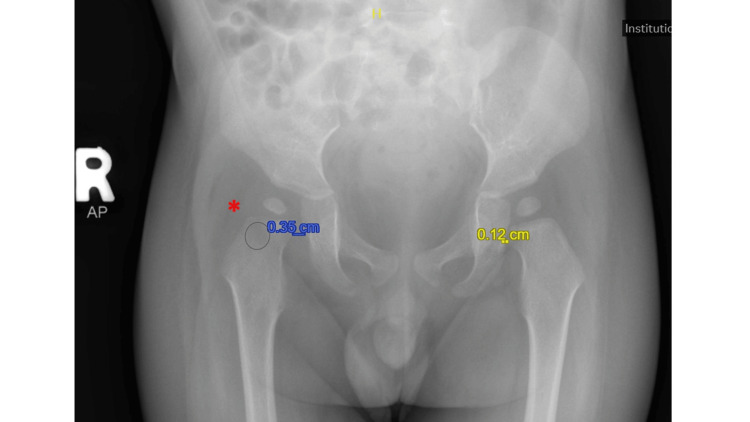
Anteroposterior radiograph of the pelvis. The image demonstrates widening of the right hip joint space, marked with red asterisk with metaphyseal rarefaction of the proximal femur (circled), suggestive of septic arthritis with associated osteomyelitis
